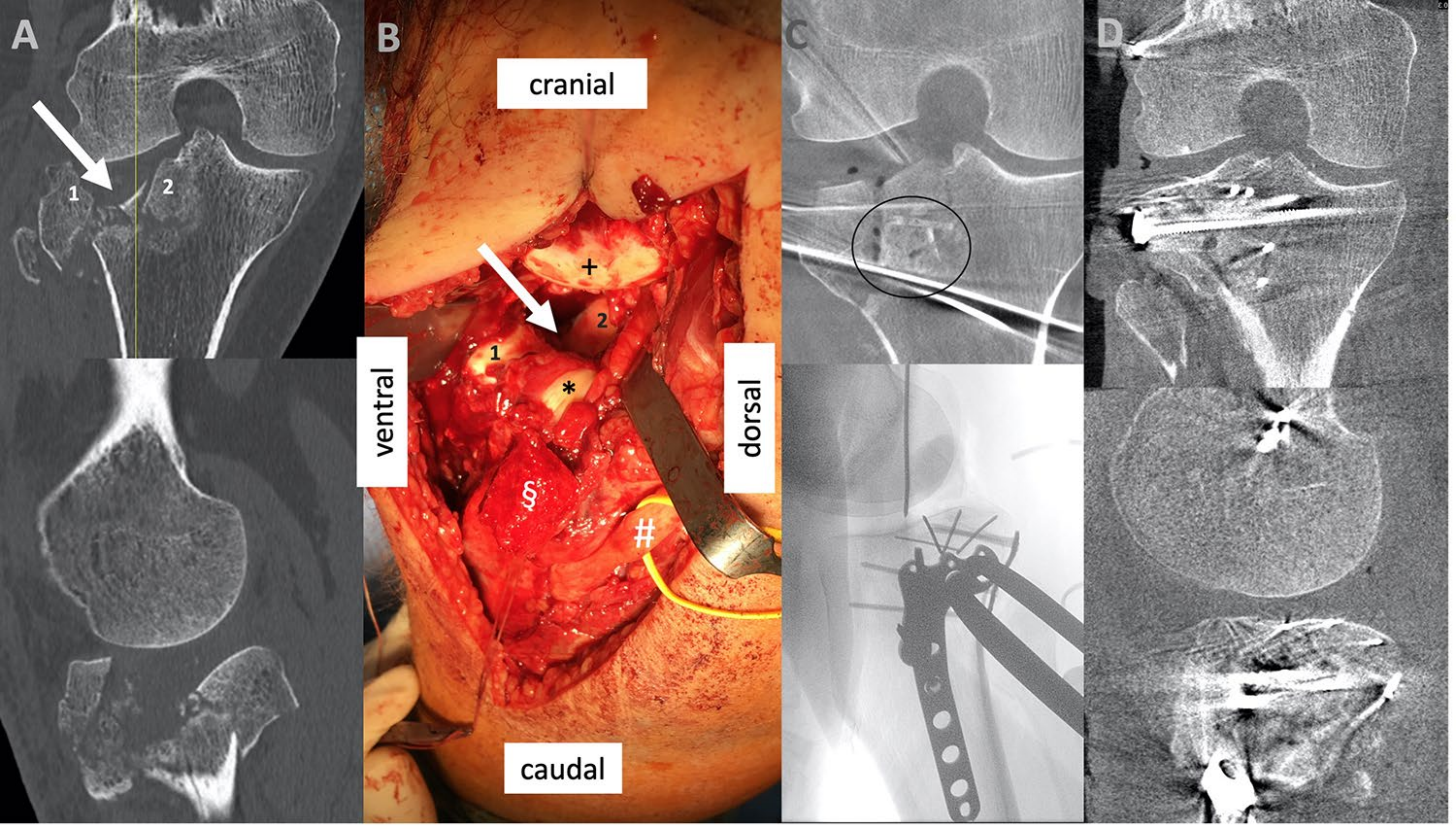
Shows preoperative and intraoperative images of the same case as shown in (Fig. 2), operated on in prone positioning, a Preoperative CT scan showing a comminuted lateral tibial plateau fracture. The arrow indicates the main depression, and two main fragments are numbered, with 1 the anterior-lateral main fragment and 2 a part of the centrally depressed main fragments. b Intraoperative situs (with # peroneal nerve, § osteotomized epicondyle fragment with * popliteus tendon and LCL attached. + Marks the lateral femoral condyles joint surface). The arrow and numbers 1 and 2 show once again the depression and the two main fragments corresponding to the markings on a; c Intraoperative coronar 3D scan after allograft implantation and first preliminary reduction attempt secured with wires, below a lateral fluoroscopic image which shows the double plate placement; d Final intraoperative 3D scan after allograft implantation and finalized ORIF
